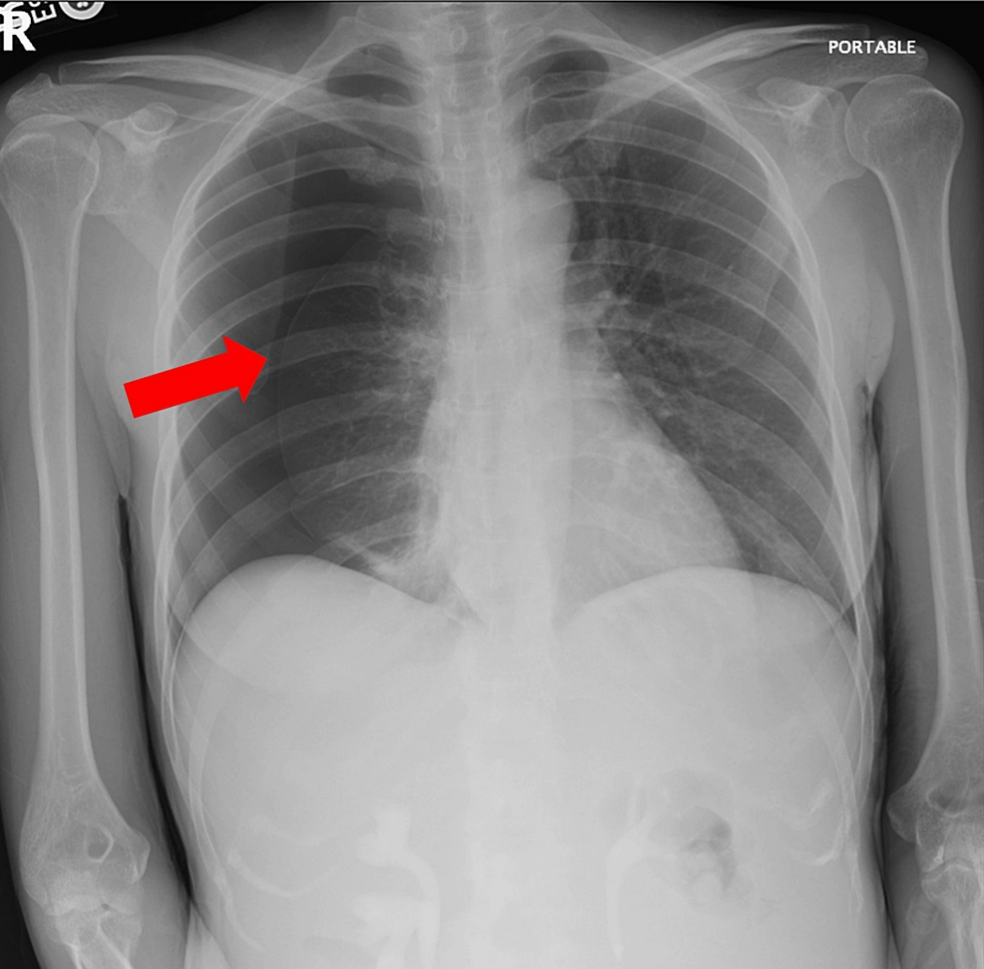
Chest X-ray showing collapsed right lung with arrow indicating the visible pleural edge line
